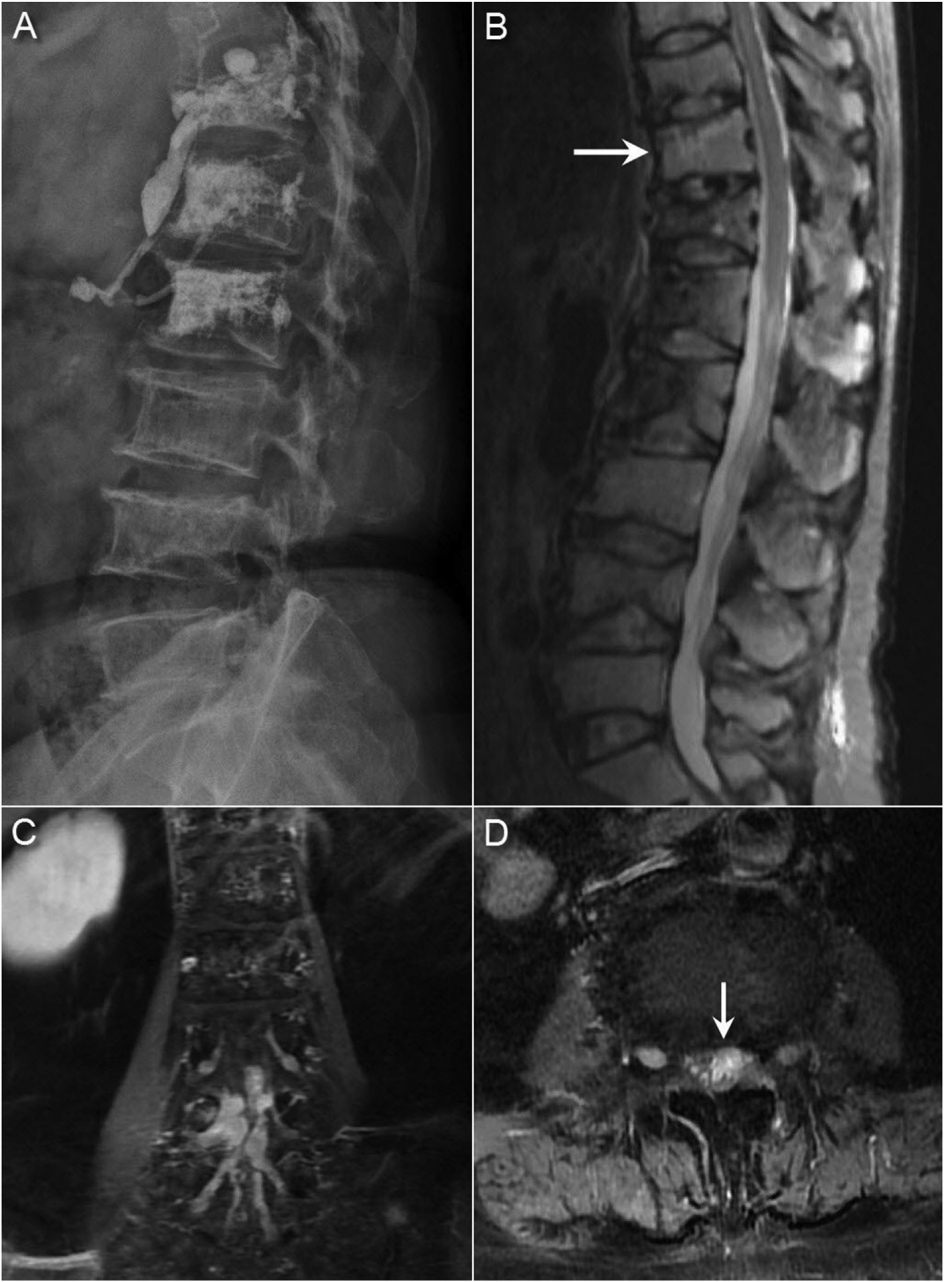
(A) Radiographs showing multiple compression fractures and a moth-eaten appearance of multiple vertebral bodies (B) Sagittal short T1 inversion recovery (STIR) sequence in the spinal MRI showing a new T11 compression fracture and multiple prior compression fractures. Coronal (C) and axial (D) T1-weighted MRI with gadolinium enhancement showing a diffuse leptomeningeal tumor spreading with extensions into the bilateral L4 and L5 neural foramen and nodularity of the cauda equina.
